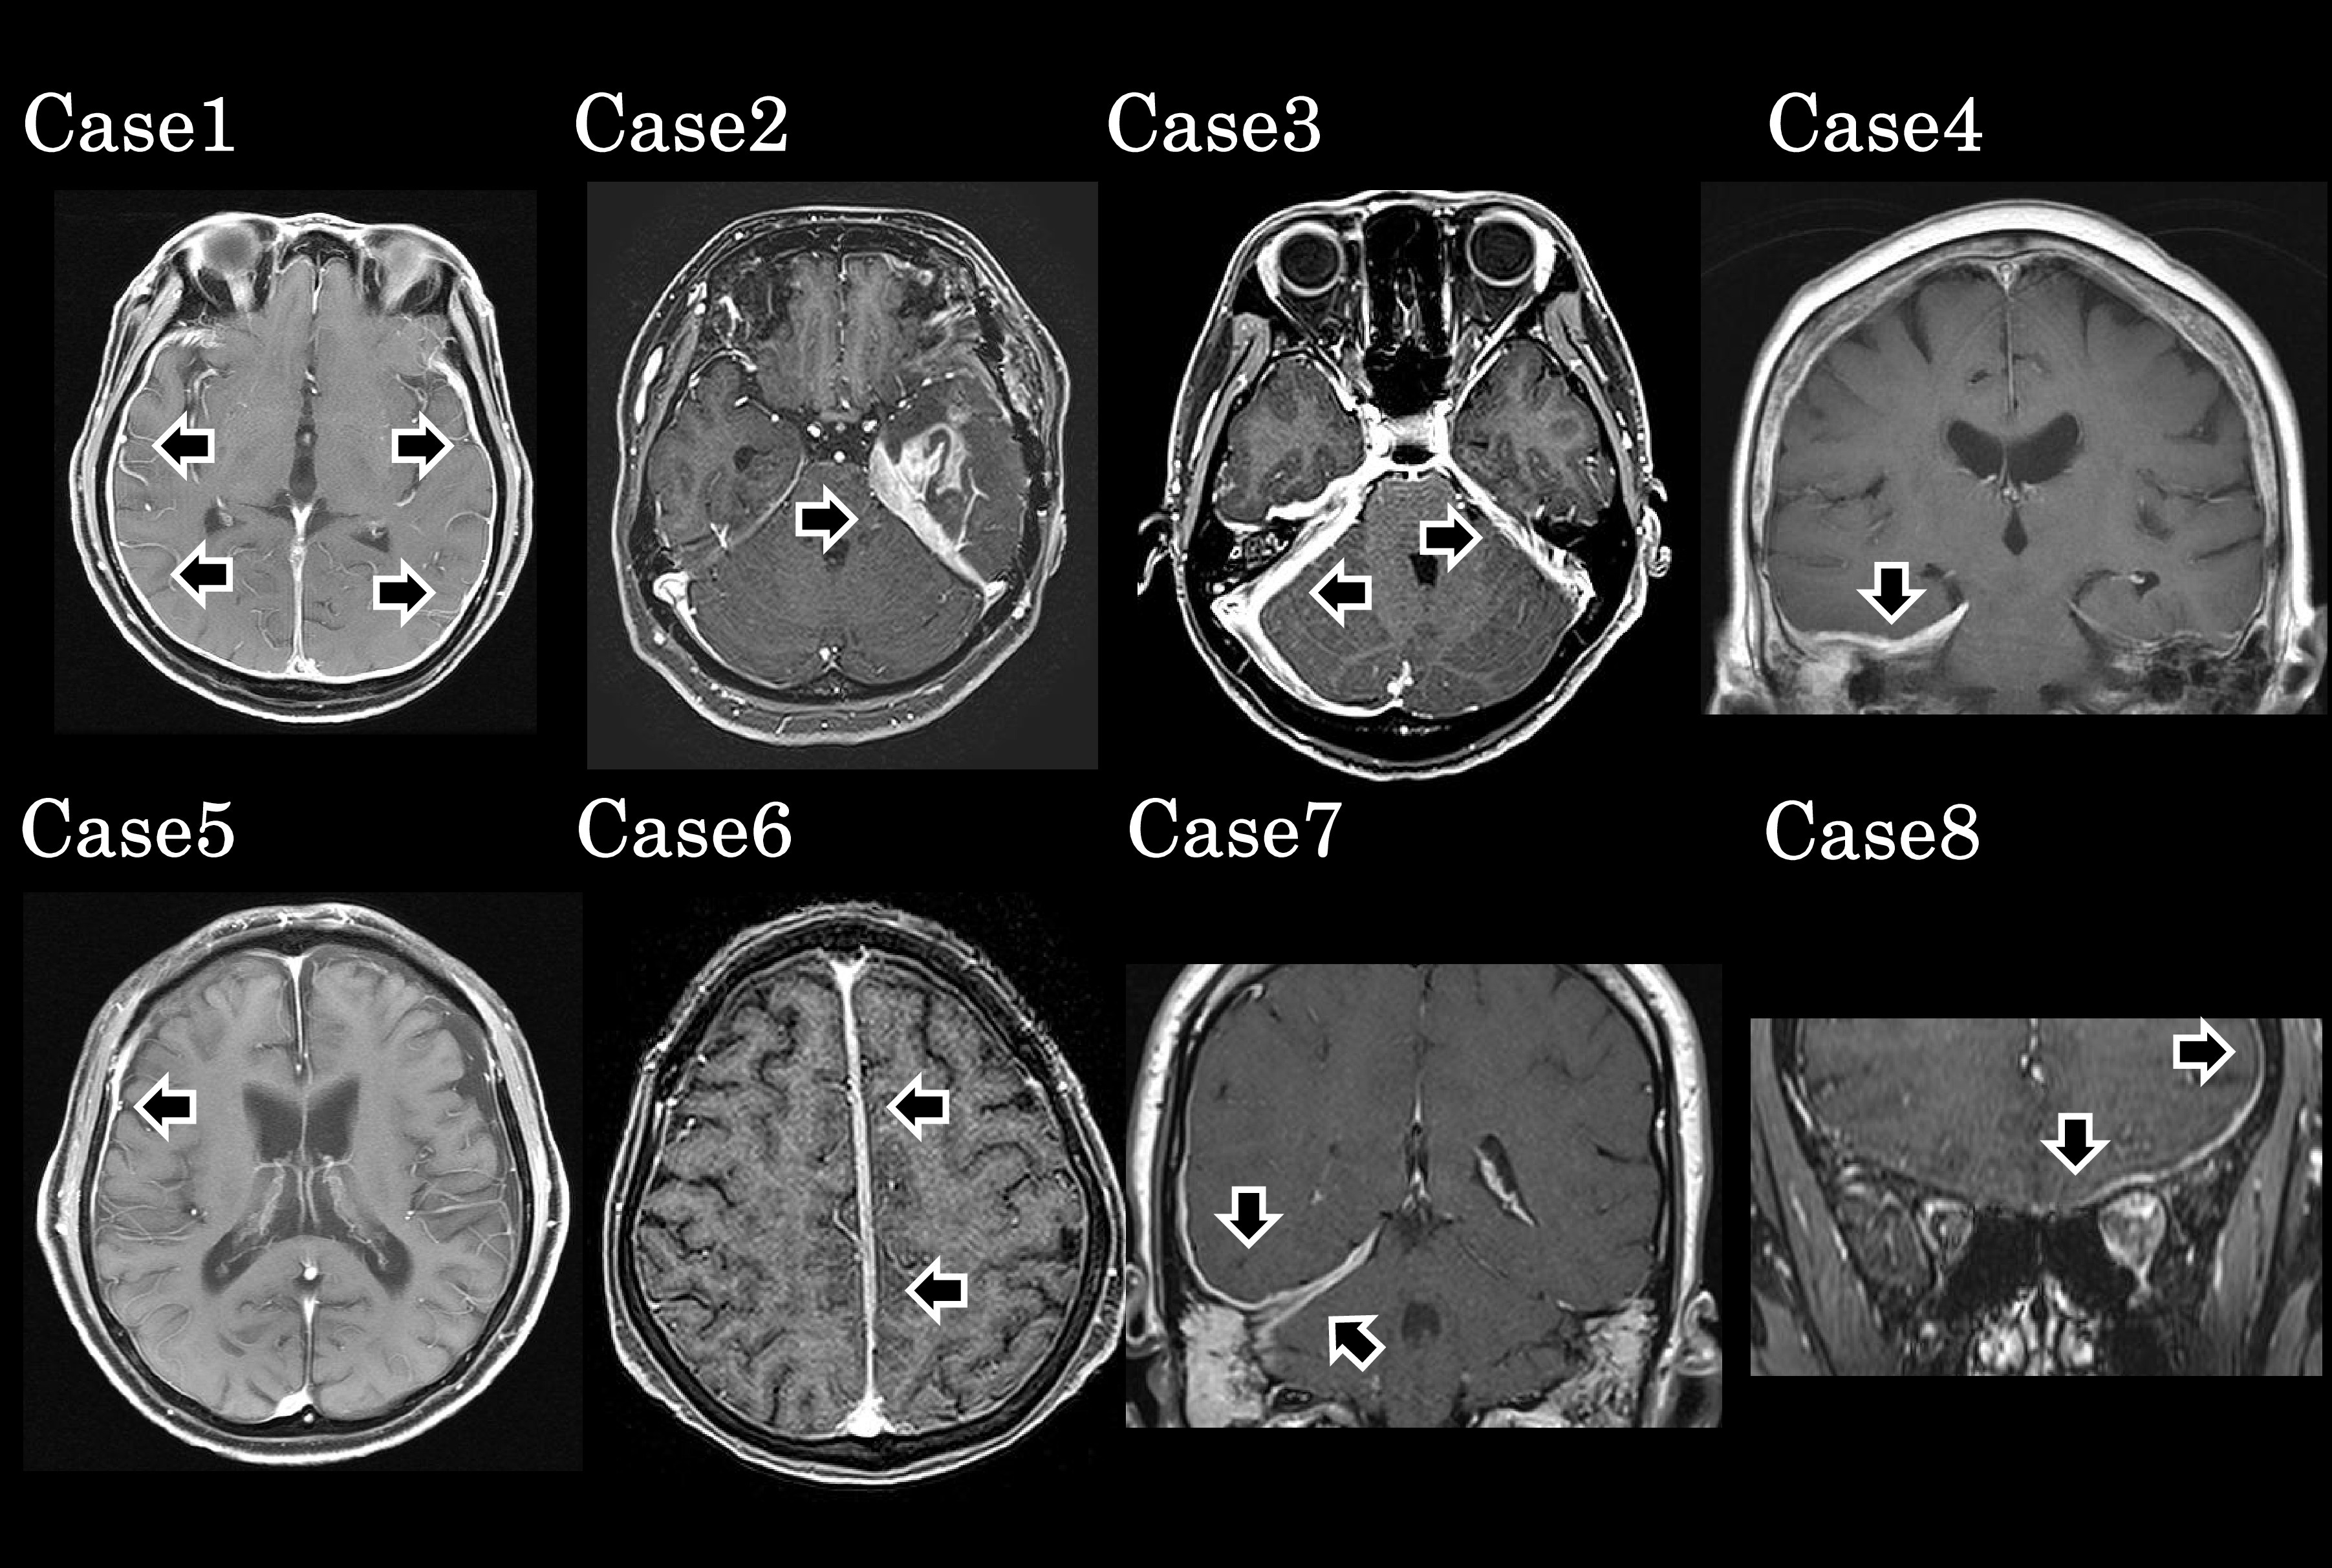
Magnetic resonance imaging findings on gadolinium-enhanced T1-weighted images of our eight patients with acute phase hypertrophic pachymeningitis.
